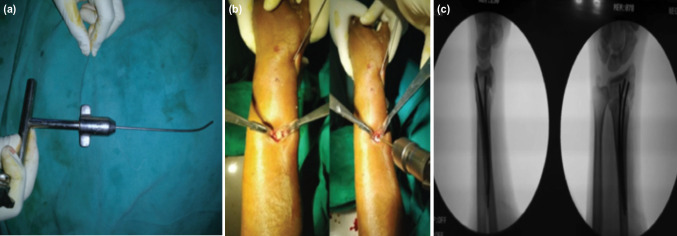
(a) Images showing a pre-bent wire prior to insertion, (b) exposure and drilling of radius for antegrade placement of wires, (c) and AP and lateral radiographs after final placement of the wires.
